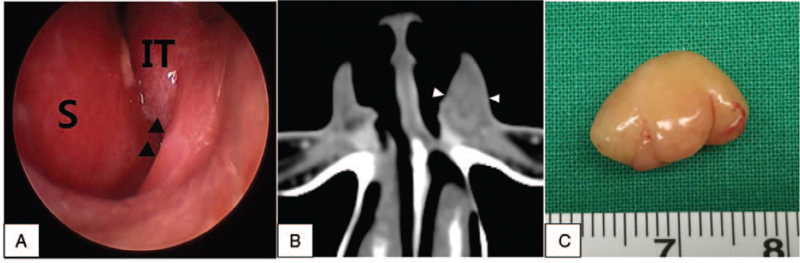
A bulging of the left vestibule is shown in the nasal endoscope (A). Computed tomography shows a mass in the left vestibule (B). The tumor is a 1.5 cm-sized yellowish mass with a smooth surface (C). IT = inferior turbinate, S = nasal septum.
